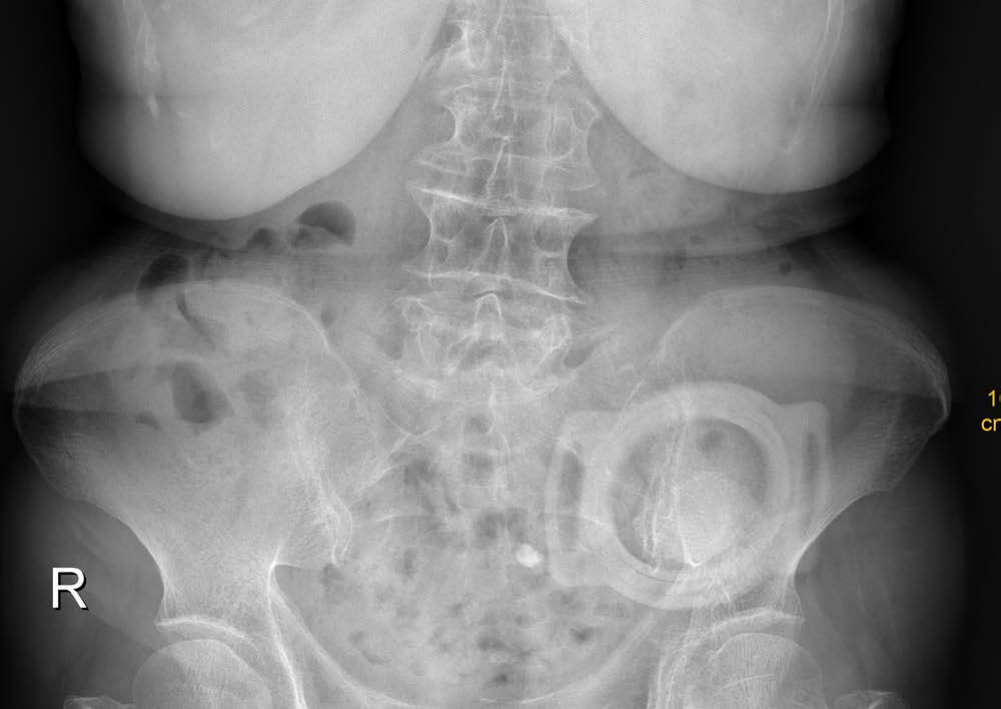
Abdominal radiograph showing no fluid levels or free air postoperatively.
